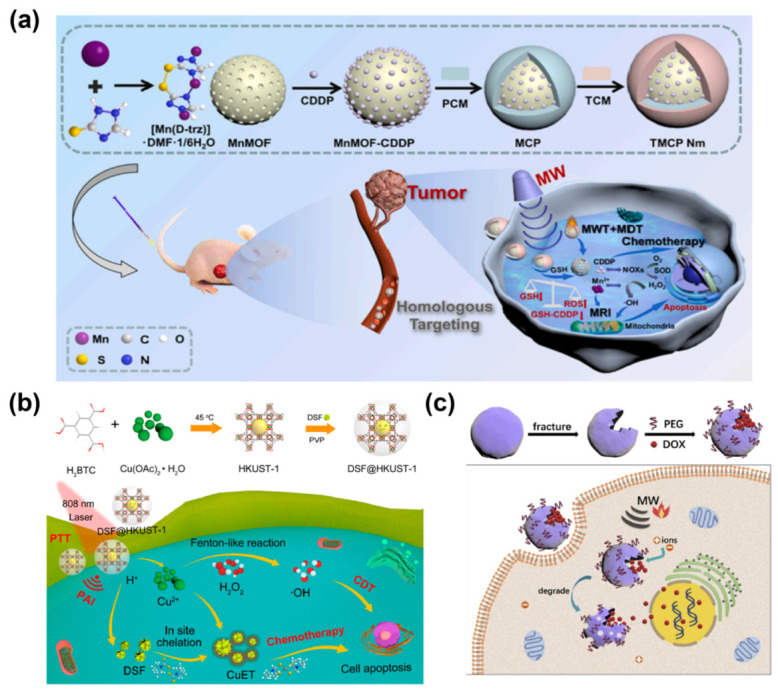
Representative MOF-based platforms for hyperthermia-chemotherapy combination therapy. (a) MnMOF-CDDP@PCM-TCM integrated cisplatin-loaded MnMOF with a phase-change material and tumor cell membrane coating, enabling thermally responsive drug release, tumor-targeted delivery, and enhanced efficacy [241]; (b) DFS@HKUST-1 underwent degradation in the acidic TME, releasing Cu^2+^ to coordinate with DSF and form the CuET complex for in situ CT, accompanied by enhanced photothermal effects [242]; (c) Construction of DOX@ZDNP@PEG by loading DOX into Zr-MOF-derived open-mouthed nano-popcorns followed by PEG surface modification for combined MWH and CT [243].
